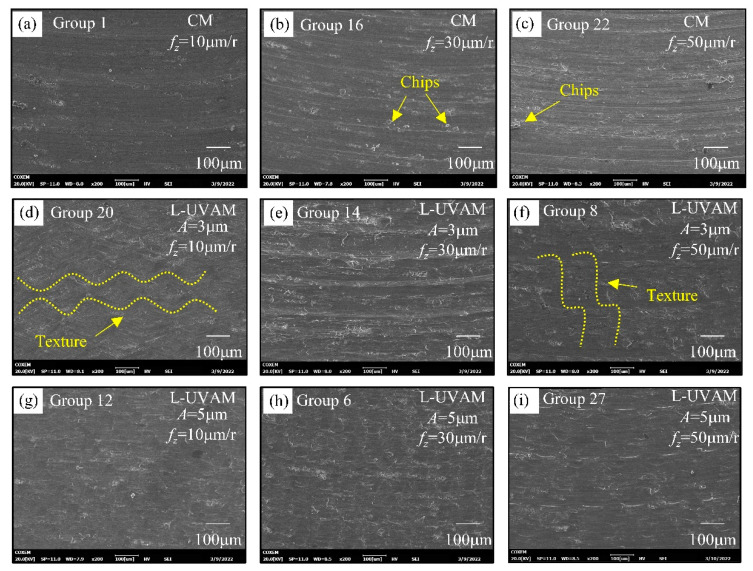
Effect of feed per tooth on the surface morphology of Conventional Milling (CM) and Longitudinal Torsion - Ultrasonic Vibration Assisted Milling (L-UVAM). (a–c) Effect of increasing feed per tooth on surface topography in CM; (d–f) Effect of ultrasonic vibration amplitude *A* = 3 μm on surface morphology; (g–i) Effect of ultrasonic vibration amplitude *A* = 5 μm on surface morphology.
